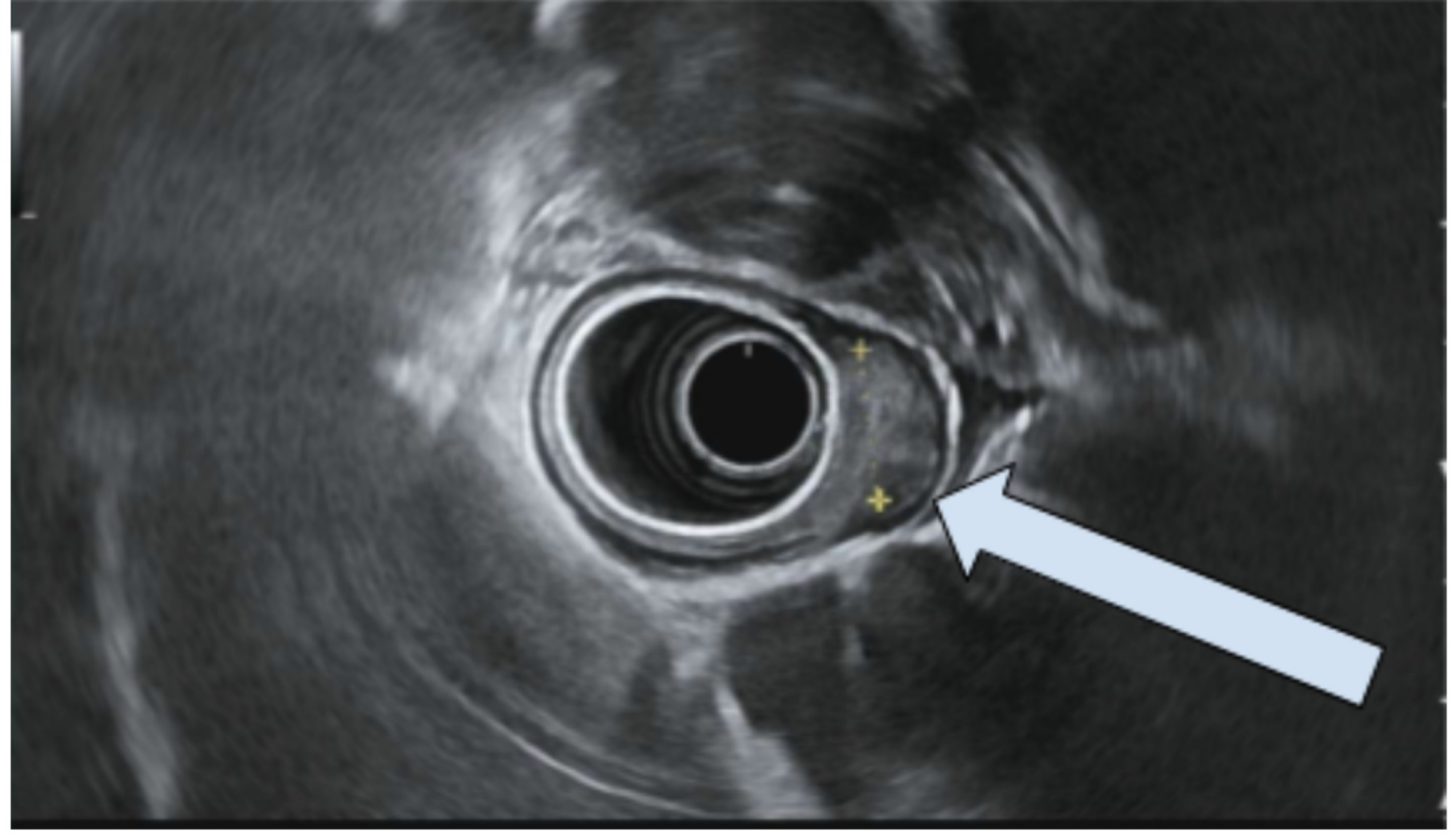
Upper endoscopic ultrasound Patchy wall thickening was visualized endosonographically in the antrum of the stomach. This appeared to primarily be due to thickening within the luminal interface/superficial mucosa (layer 1) and deep mucosa (layer 2). No intramural lesions were visualized underneath the mucosa.
